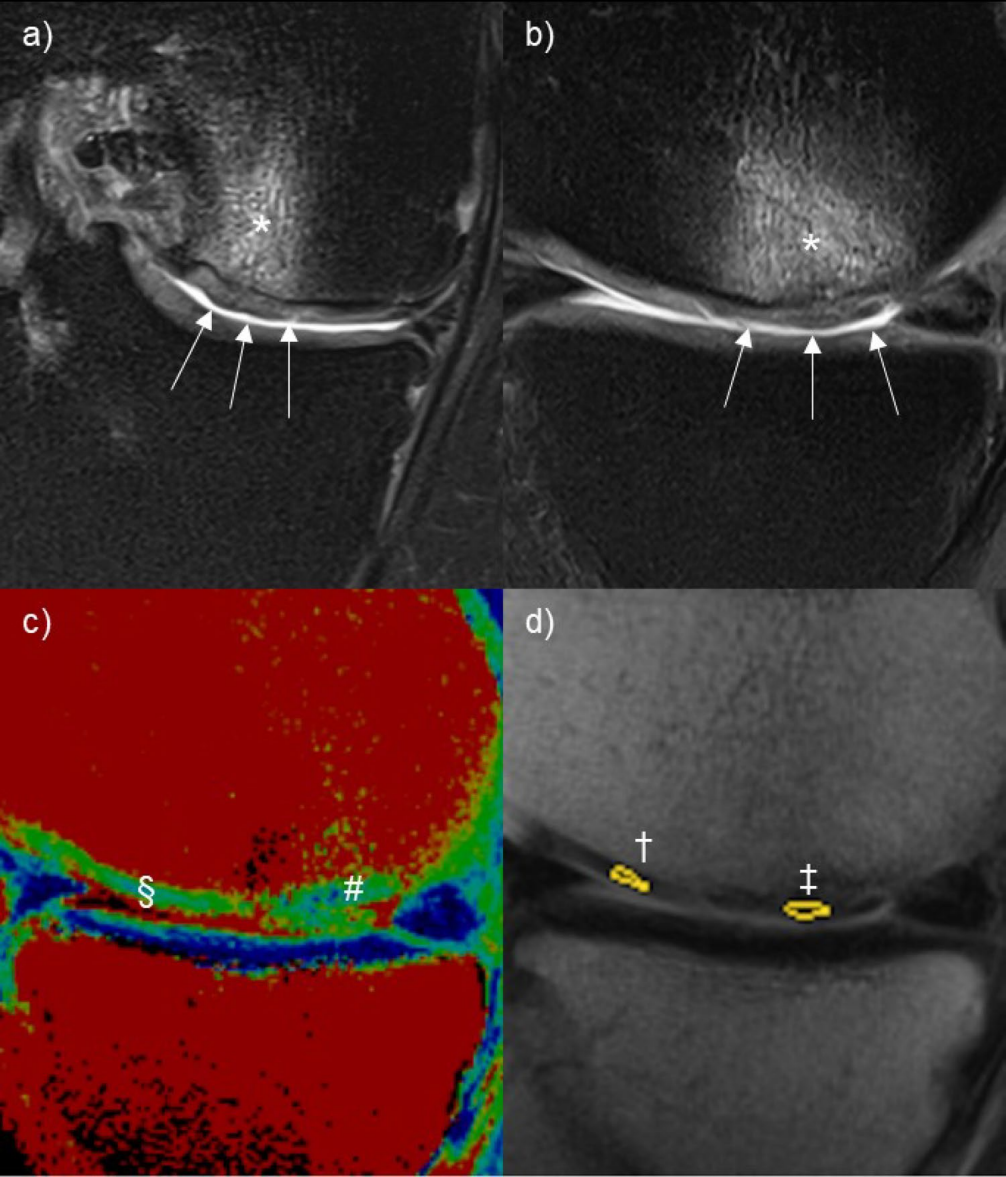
Postoperative MRI of case 3. a Coronal Proton Density Turbo Spin Echo sequences (PD TSE) showing the transplanted cartilage (arrows) and associated bone marrow oedema (*). b Sagittal PD TSE sequences showing the transplanted cartilage (arrows) and associated bone marrow oedema (*). c Sagittal T2 Mapping sequence showing colour coding of transplanted cartilage (^#^) and adjacent healthy hyaline cartilage (^§^). d Sagittal T2 Mapping sequence without colour code of transplanted cartilage (^‡^) and adjacent healthy hyaline cartilage (^†^), yellow circles mark the respective region of interest (ROI) in which the relevant T2 relaxation time of included cartilage tissue was measured to calculate the T2-Index
